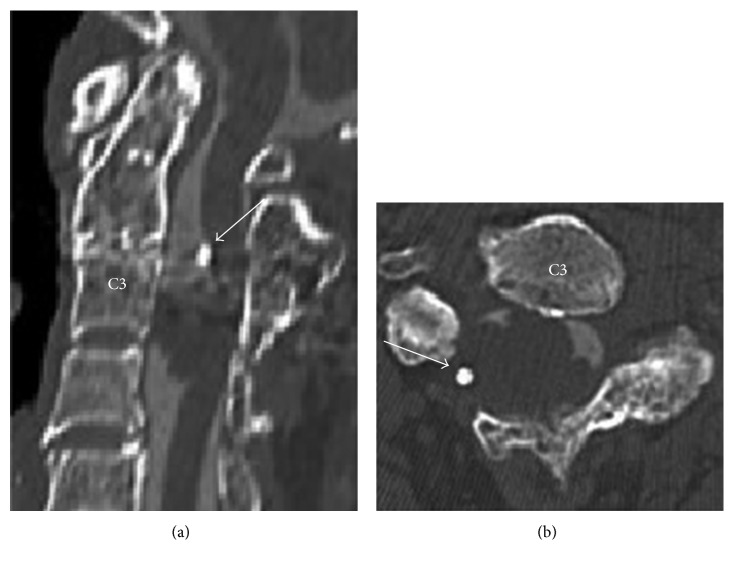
Computed tomographic myelography also showing clearly delineated intradural extramedullary mass and the previously placed shunting tube (arrows). (a) Sagittal image. (b) Axial image.
